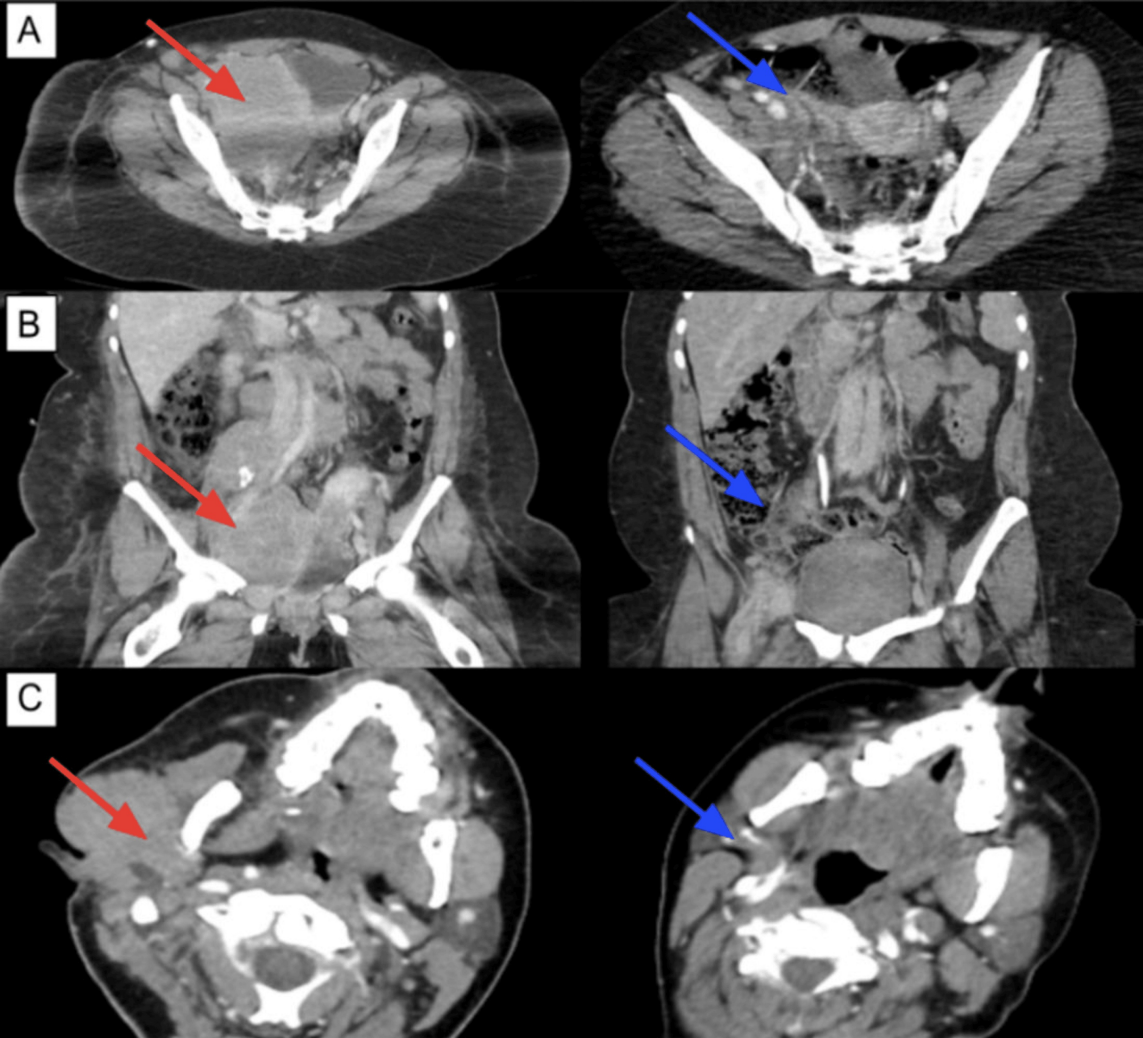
(A) Axial CT images of pelvic lymphoma before (red arrow) and after (blue arrow) two cycles of treatment with R-EPOCH. (B) Coronal CT images of paraaortic and aortocaval lymphoma before (red arrow) and after (blue arrow) treatment. (C) Axial CT images of cheek lymphoma before (red arrow) and after (blue arrow) treatment.
